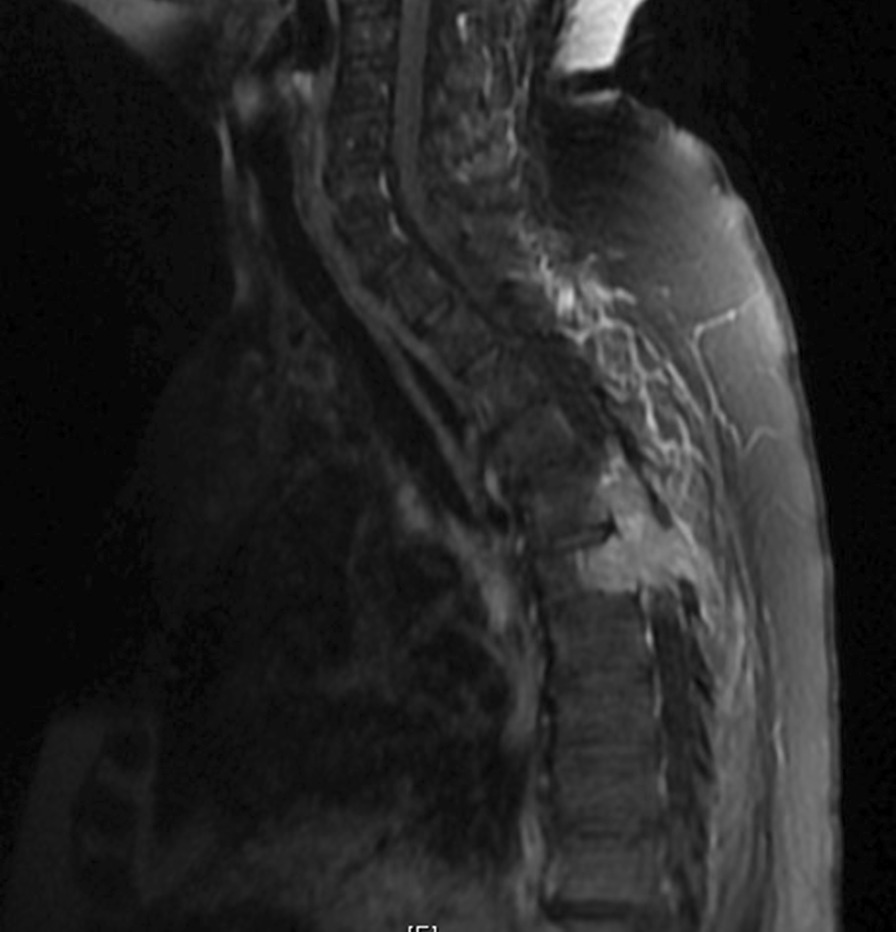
Baseline T1 (left) MRI sequences showing soft tissue metastasis at T6 with epidural soft tissue component and edema and extension to spinal cord in Patient A
